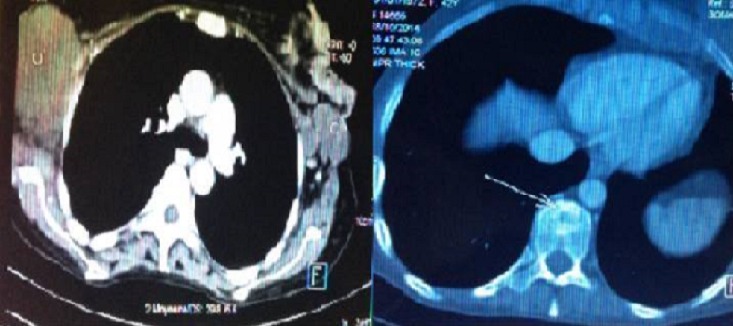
CT scan showed a contralateral breast progression and apparition bone metastatsis at D10
